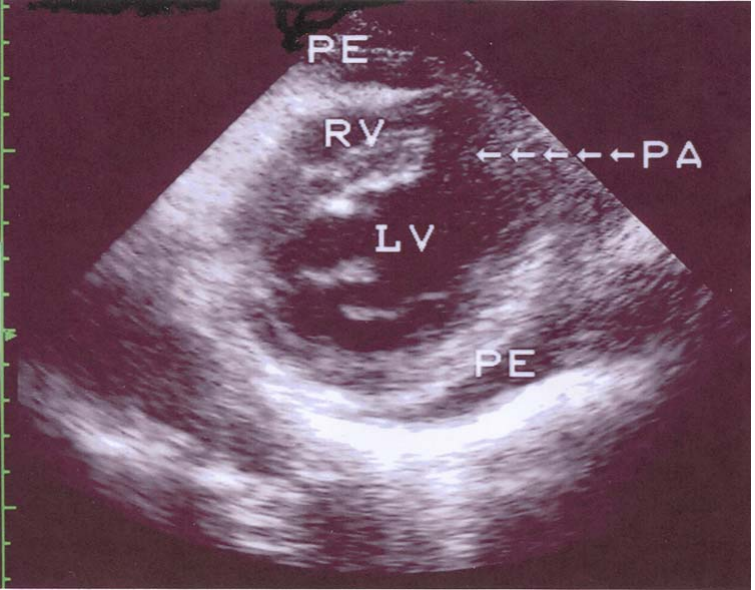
Modified left parasternal short axis view that shows a discontinuity of the apical part of the interventricular septum and the LV apex, a communication between the left and the right ventricle, and a small cavity contained by epicardium (pseudoaneurysm) through a narrow neck. (LV: left ventricle, RV: right ventricle, PA: pseudoaneurysm, PE: pericardial effusion).
